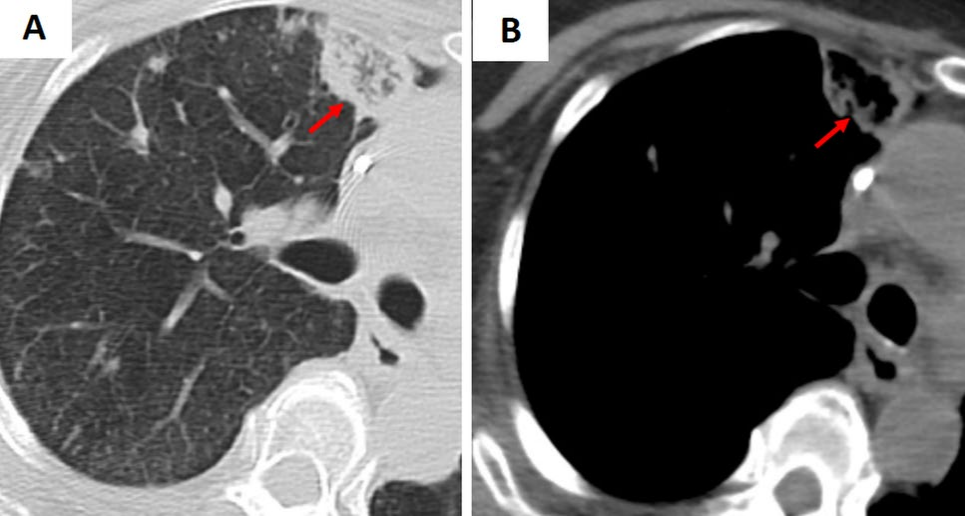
Reverse halo (atoll) sign in a patient with metastatic malignant melanoma. A, B Chest CT images demonstrate an irregular right upper lobe mass with the reverse halo (atoll) sign (arrows). Metastasis was confirmed via biopsy. Note the presence of additional pulmonary nodules suggestive of metastasis. The patient also had multiple hepatic metastases (not shown)
